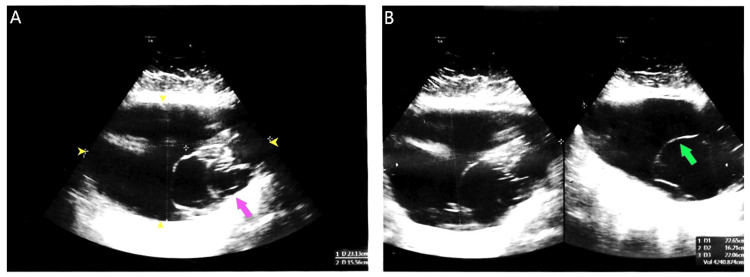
Ultrasonography of the abdomen and pelvis showing A) 23.1x15.5x22.0 cm complex multiloculated cystic lesion (yellow arrowheads) with a thick outer wall (purple arrow) and B) multiple internal septations (green arrow).
